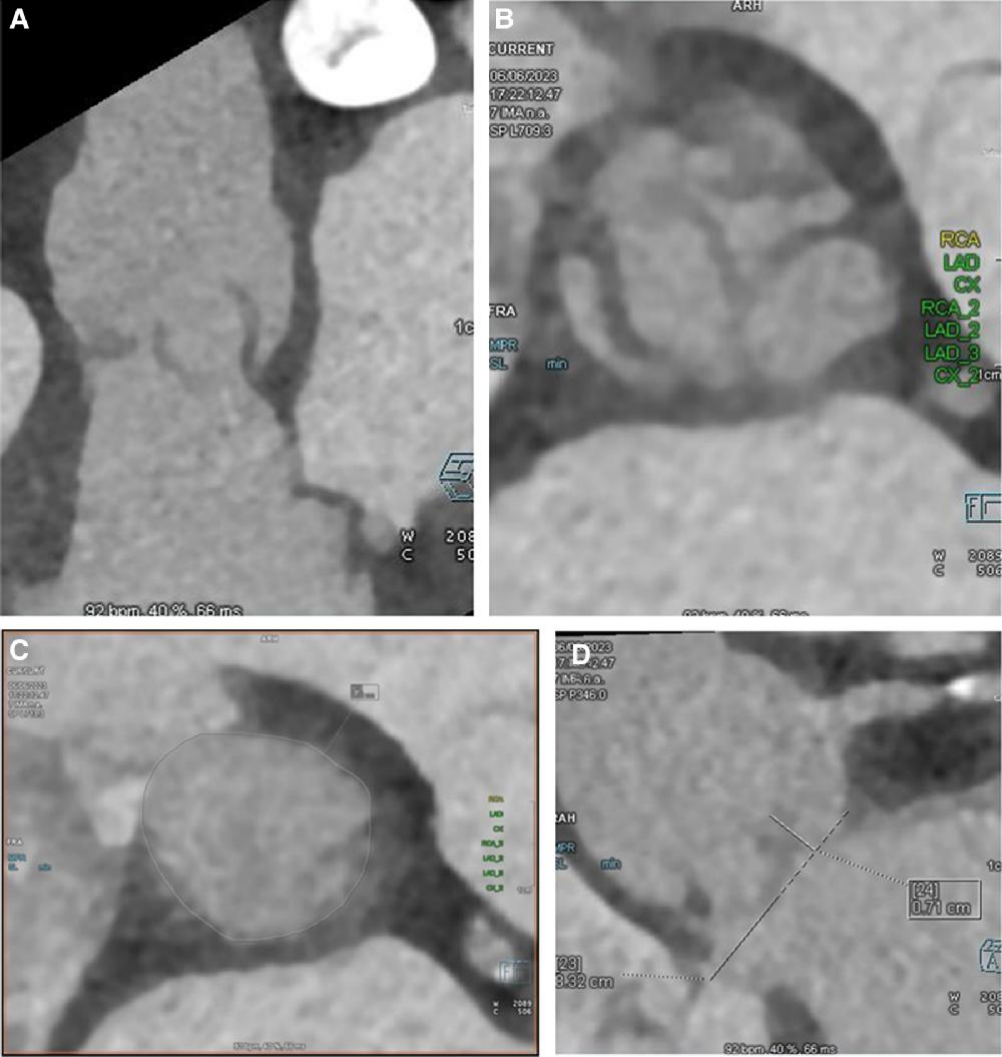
Computed tomography demonstrating absence of aortic valve calcification (*A* and *B*). (*C*) Neo-annulus measured 660 mm^2^; (*D*) left main coronary height 7.1 mm.
